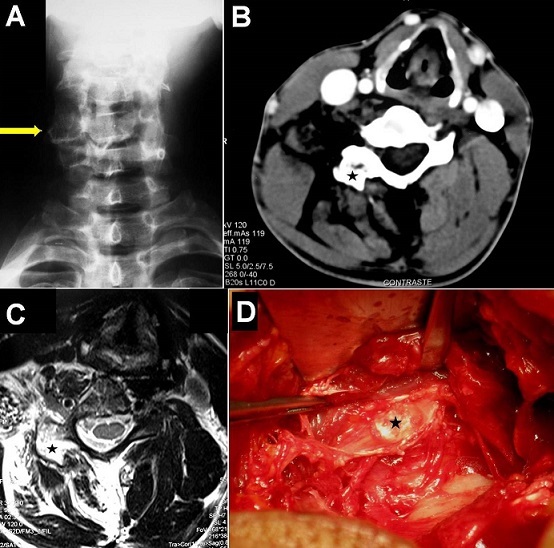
(A) Antero-posterior cervical radiograph showing the scoliosis with right unilateral C5-C6 facet joint hypertrophy (arrow). (B and C) Axial CT-scan and T2-weighted MR imaging demonstrating the osseous well-circumscribed tumor (star) in the right C6 articular process and diffuse amyotrophia of the neck musculature on the right side without nerve root or spinal cord compression. (D) Intraoperative photograph of the calcified cartilaginous tumor (star) before removal
